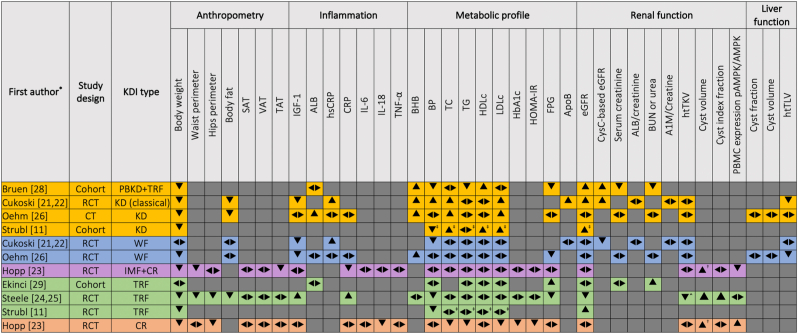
SWiM (qualitative synthesis) of the results of studies assessing the efficacy of KDIs in patients with ADPKD. A1M: Alpha-1-microglobulin; ADPKD, autosomal dominant polycystic kidney disease; ALB: albumin; AMPK: AMP-activated protein kinase; ApoB: apolipoprotein B; BHB: beta-hydroxybutyrate; BP: blood pressure; BUN: blood urea nitrogen; CRP: C-reactive pro-tein; CysC: systatin C; CT: clinical trial; FPG: fasting plasma glucose; eGFR: estimated glomerular filtration rate; HbA1c: glycosylated haemoglobin; HDLc: high density lipoprotein cholesterol; HOMA-IR: Homeostatic Model Assessment for Insulin Resistance; hsCRP: high-sensitivity C-reactive protein; htTKV: height adjusted TKV; IGF-1: insulin-growth factor 1; IMF: intermittent fasting; IL-6: interleukin 6; IL-18: interleukin 18; KD: ketogenic diet; KDI: ketogenic dietary inter-vention; LDLc: low-density lipoprotein cholesterol; NR: not reported; pAMPK: phosphorylated AMP-activated protein kinase; PBKD: plant-based ketogenic diet; PCMC: peripheral blood mon-onuclear cell; RCT: randomized controlled trial; SAT: subcutaneous adipose tissue; TAT: total adipose tissue; TC: total cholesterol; TG: triglycerides; TKV: total kidney volume; TLV: total liver volume; TNF-α: tumor necrosis factor α; TRF: time-restricted feeding; VAT: visceral adipose tis-sue; WF: water fasting. * Testa [30] provided individual patient changes instead of average sam-ple results; ‡ self-reported. † height corrected.
